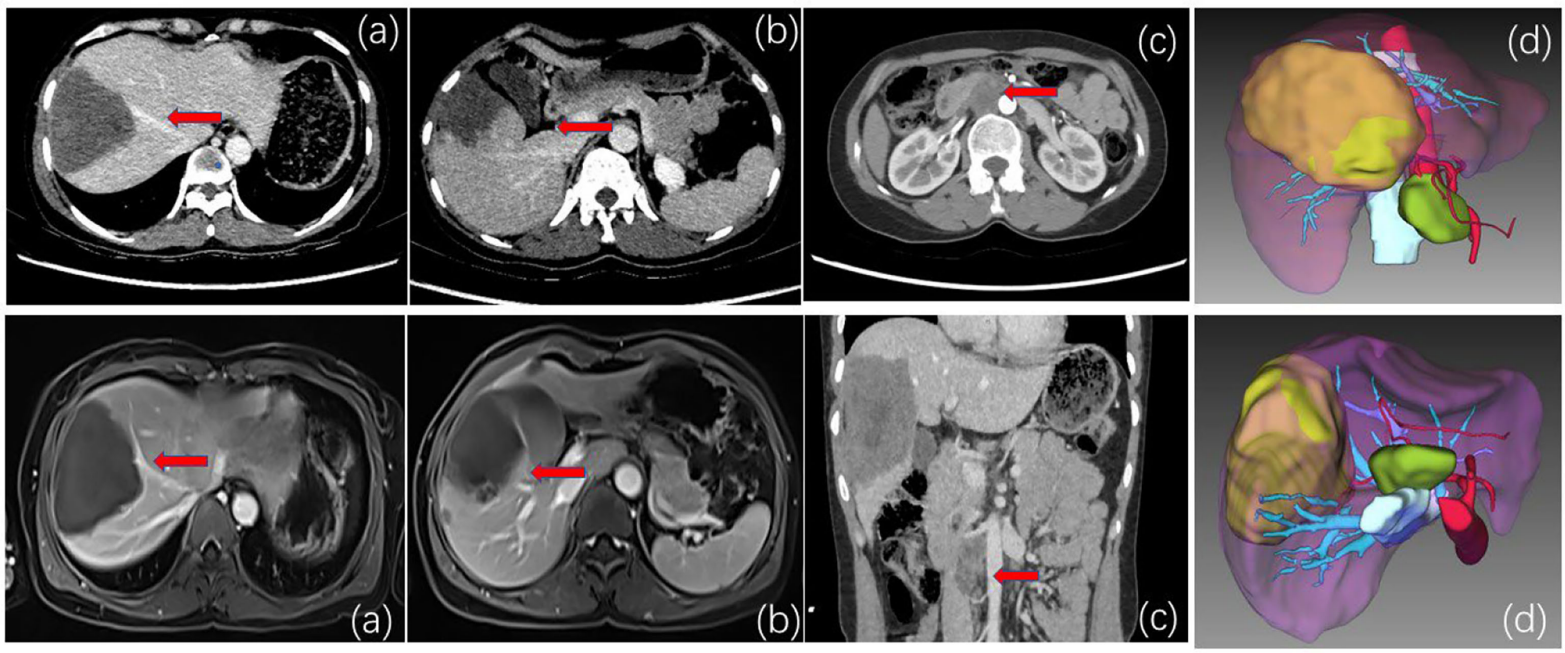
(A) CT and MRI venous phase. The red arrow indicates that the middle hepatic vein is close to the lesion and is considered to be invaded. (B) CT and MRI delayed phase. The red arrow indicates that the right anterior branch of the portal vein is considered to be invaded. (C) CT and MRI. The red arrow indicates inferior vena cava-para-abdominal aortic swelling. Large lymph nodes are approximately 2*2 cm in diameter. The lesions are low signal, and no enhancement is seen after enhancement. Thus, lymph node metastasis of alveolar echinococcosis was considered. (D) Preoperative three-dimensional CT and imaging showed enlarged lymph nodes in the inferior vena cava-abdominal aorta. Considered secondary lymph node metastasis of alveolar echinococcosis.
